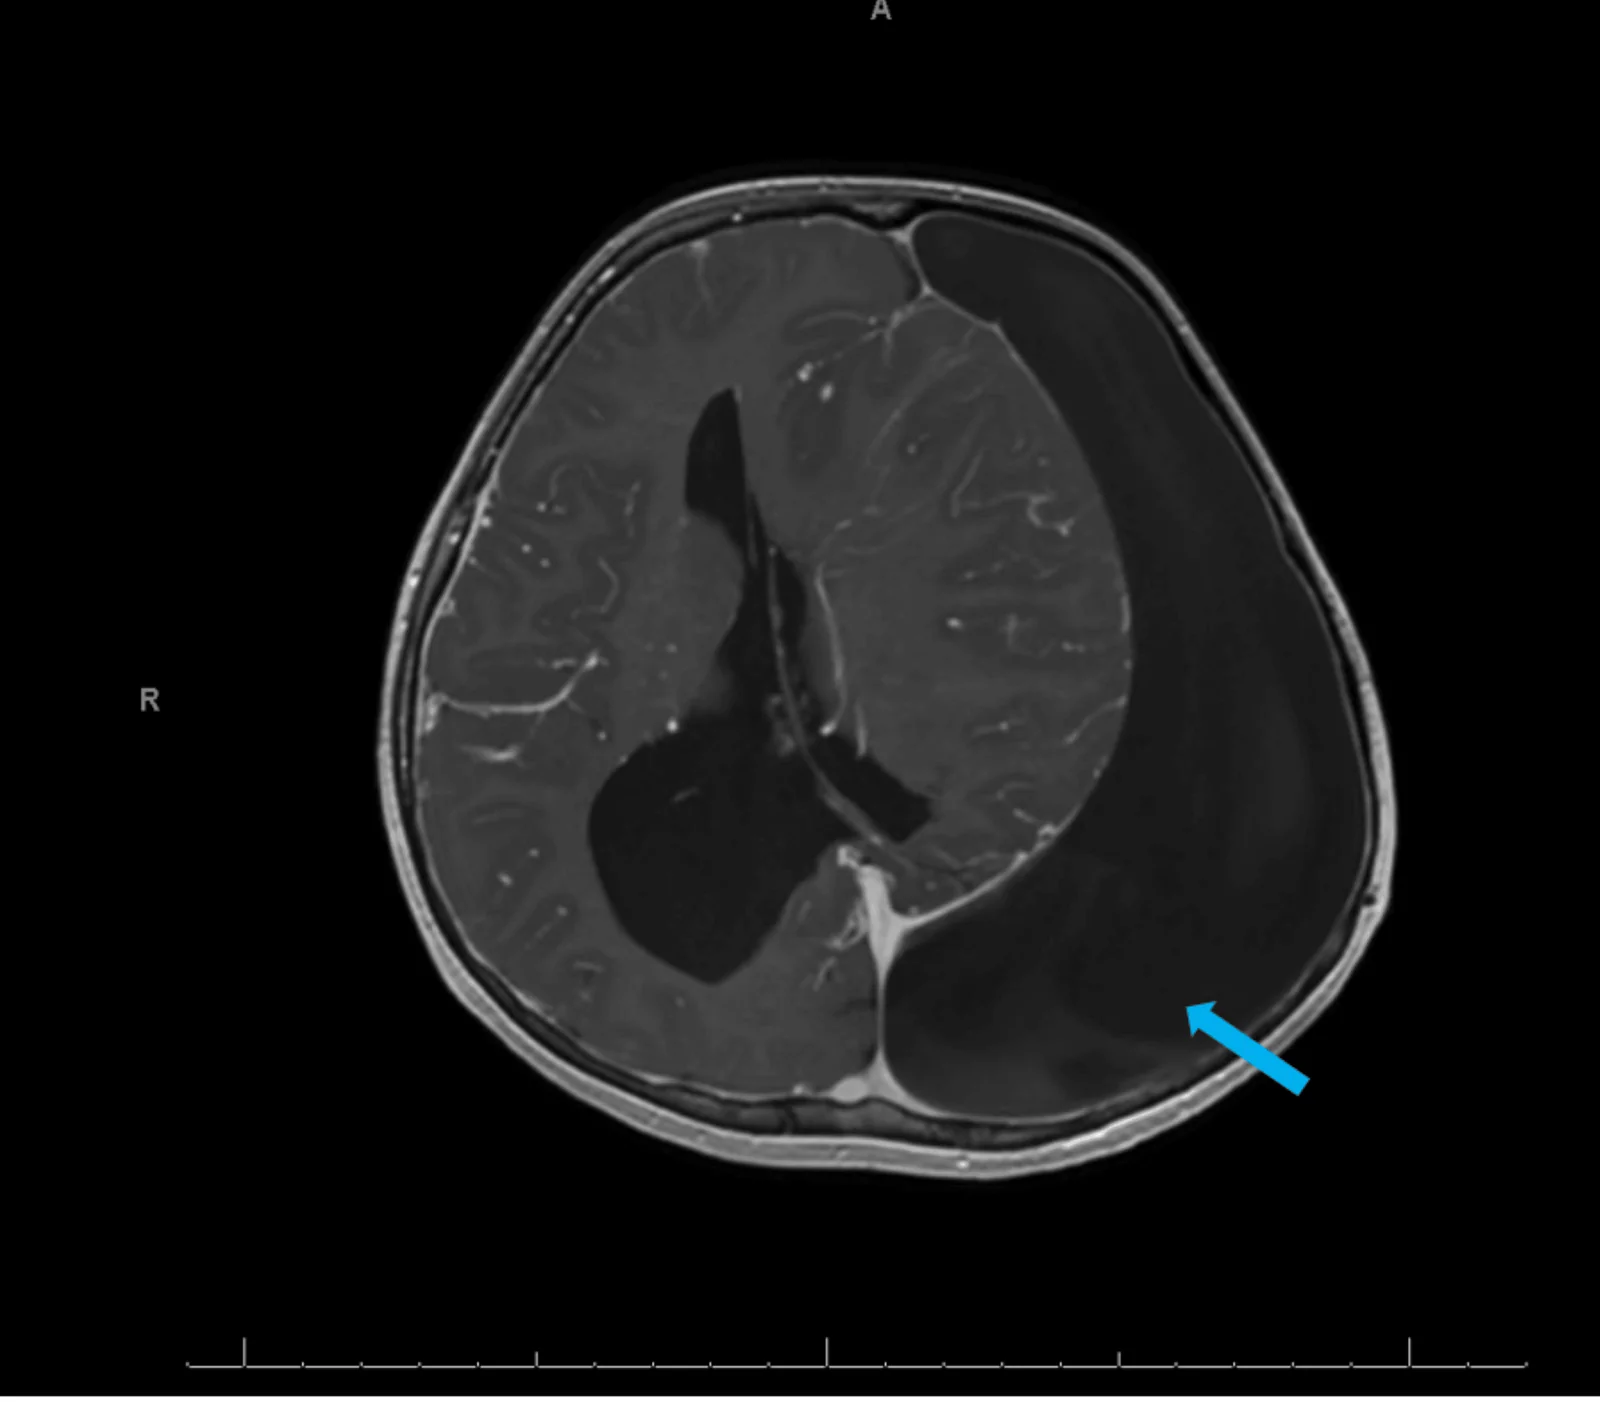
Most recent MRI (T1 post-contrast). Stable post-operative changes with resolution of the prior hematoma and a persistent but stable extra-axial fluid collection on the same side of resection (indicated by the blue arrow), with stable ventriculomegaly. No evidence of recurrent tumor noted.
